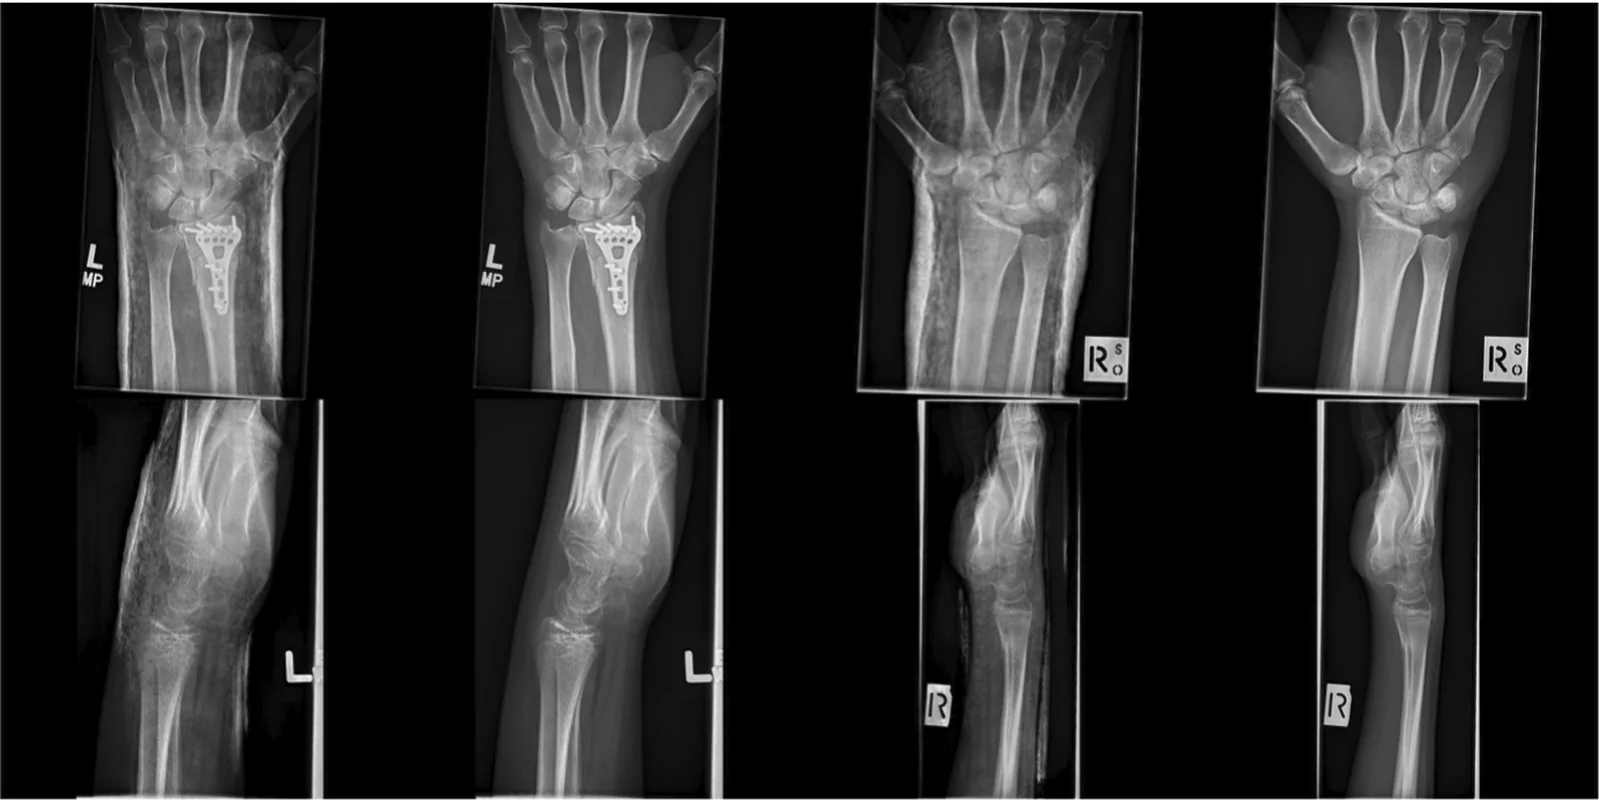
Representative sample of synthetic paired wrist X-rays, showing CycleGAN-generated cast images (left) and corresponding original cast-free images (right)
